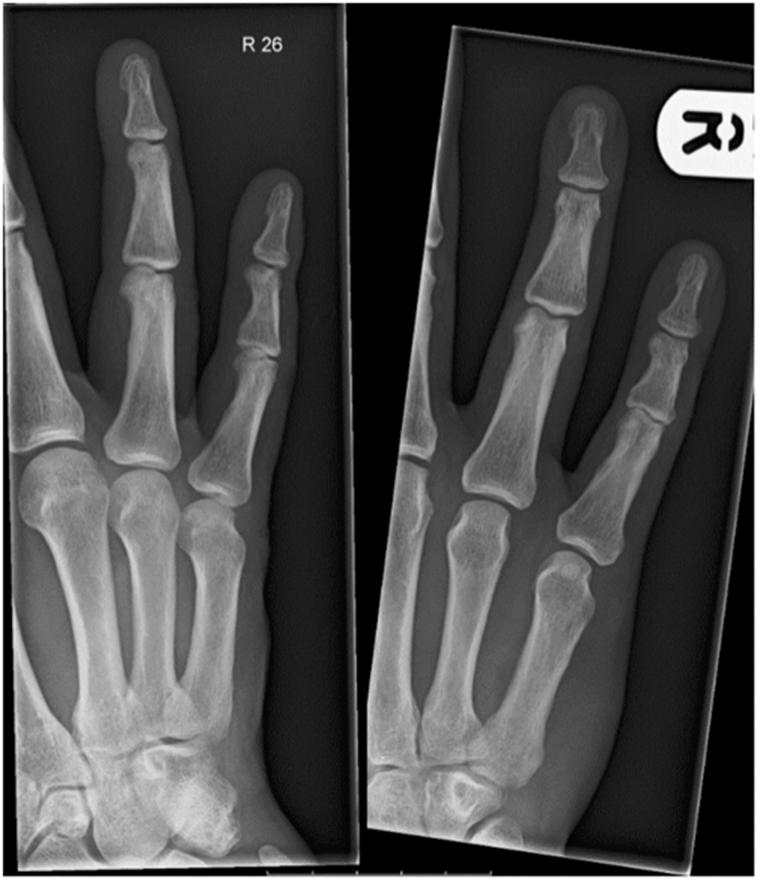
Radiograph right hand showing no bony irregularities.
